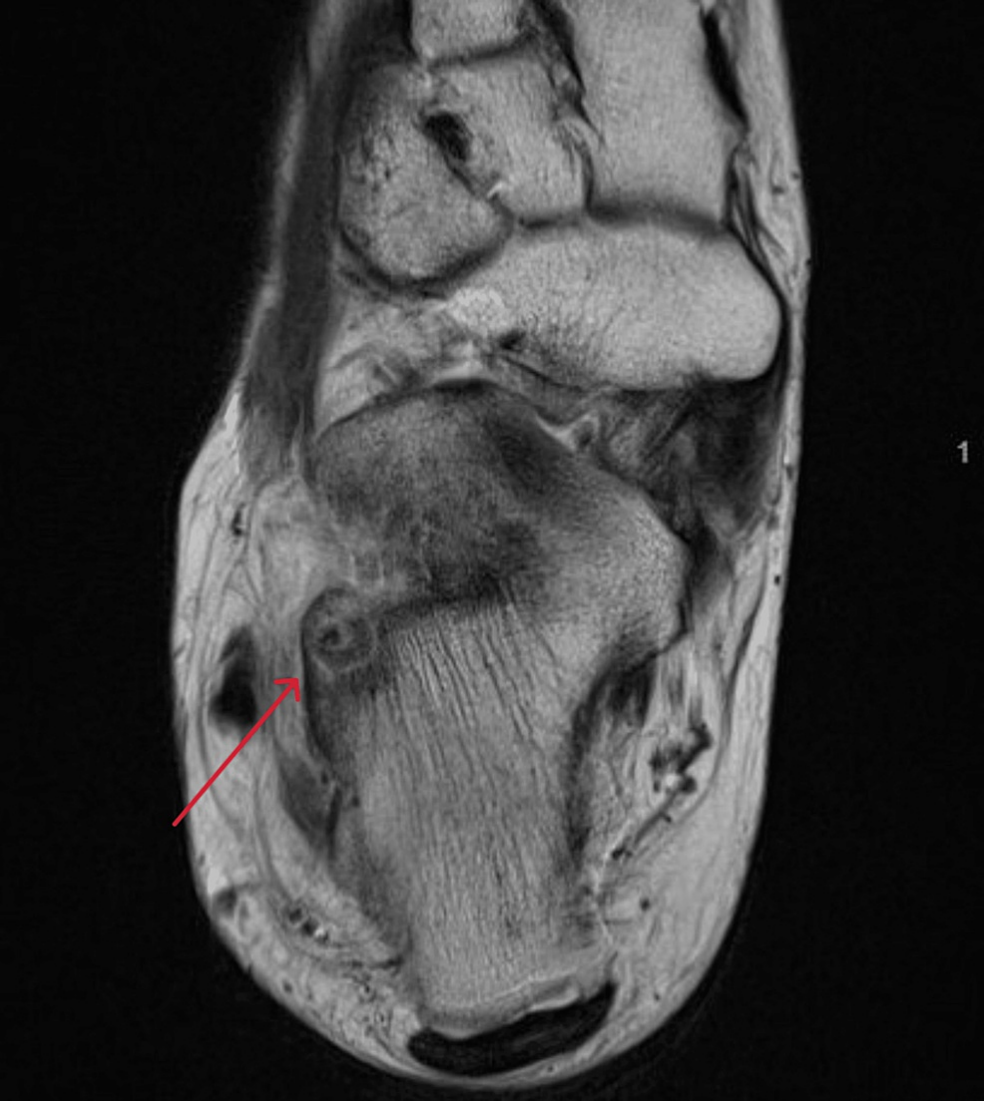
Axial view MRI T1 window of the right ankle at the time of presentation MRI: magnetic resonance imaging
